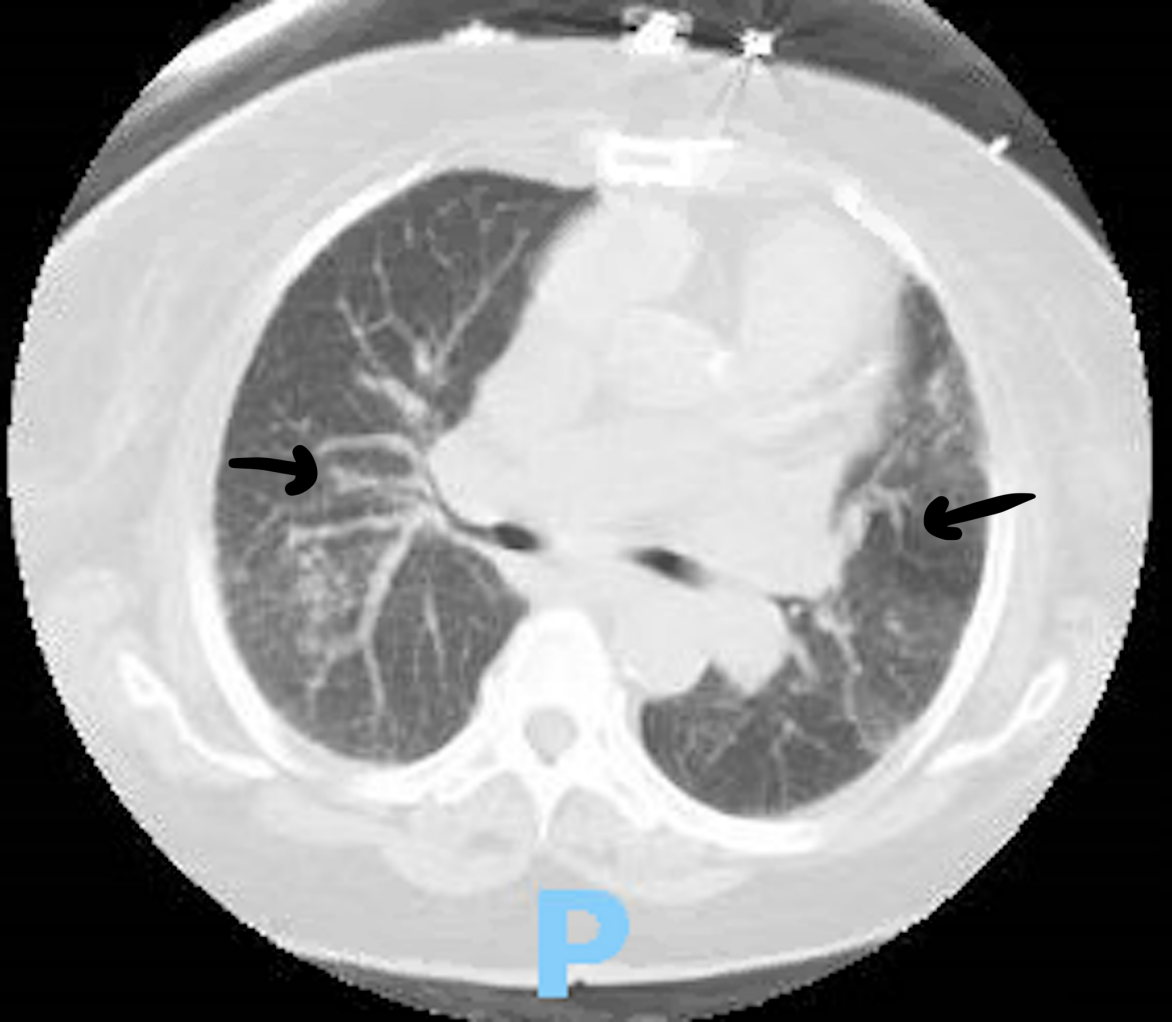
The figure from the CT scan shows bilateral interstitial opacities. Arrows pointing towards characteristic findings of interstitial lung disease.
